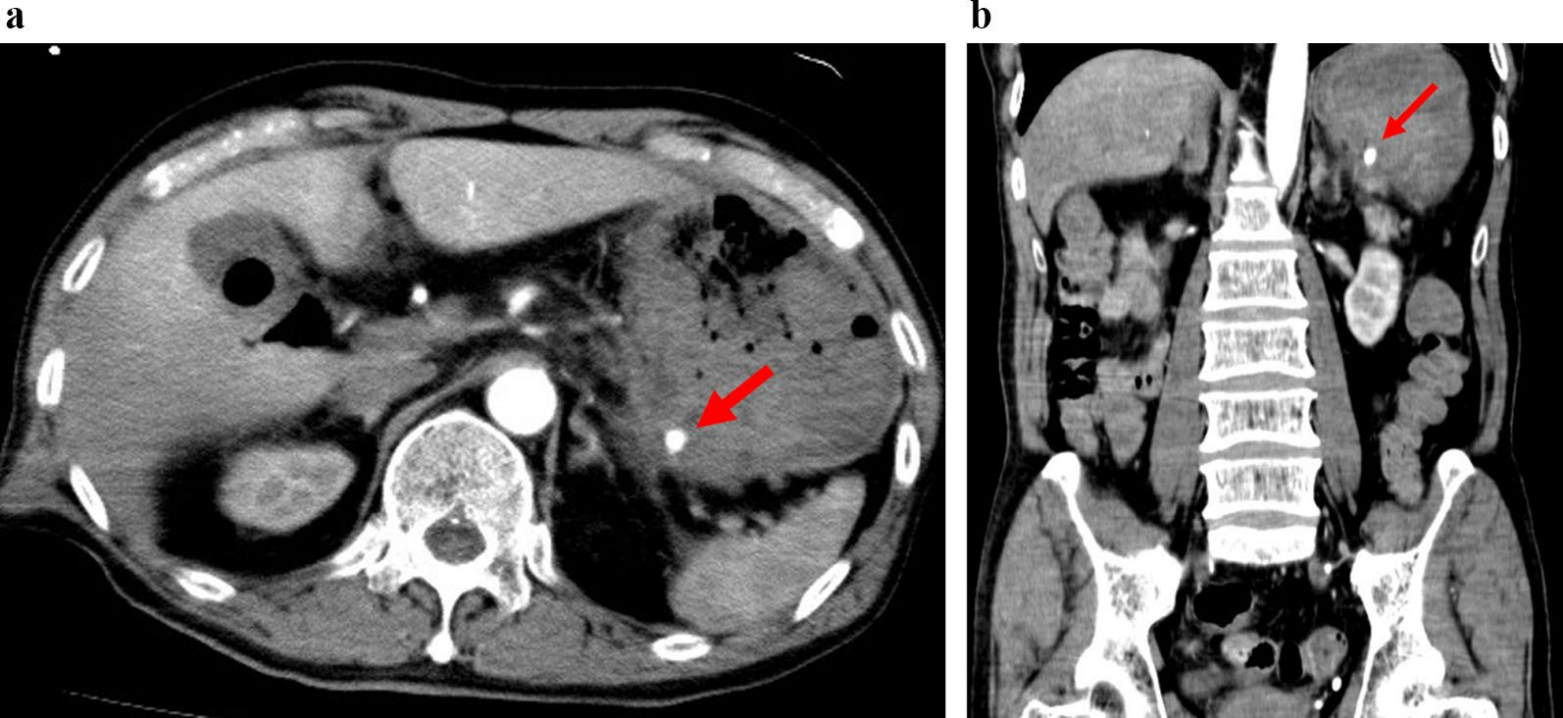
a Axial computed tomography showing a splenic artery aneurysm (red arrow) that ruptured into the stomach on admission. b Coronal computed tomography showing a splenic artery aneurysm (red arrow) that ruptured into the stomach on admission
